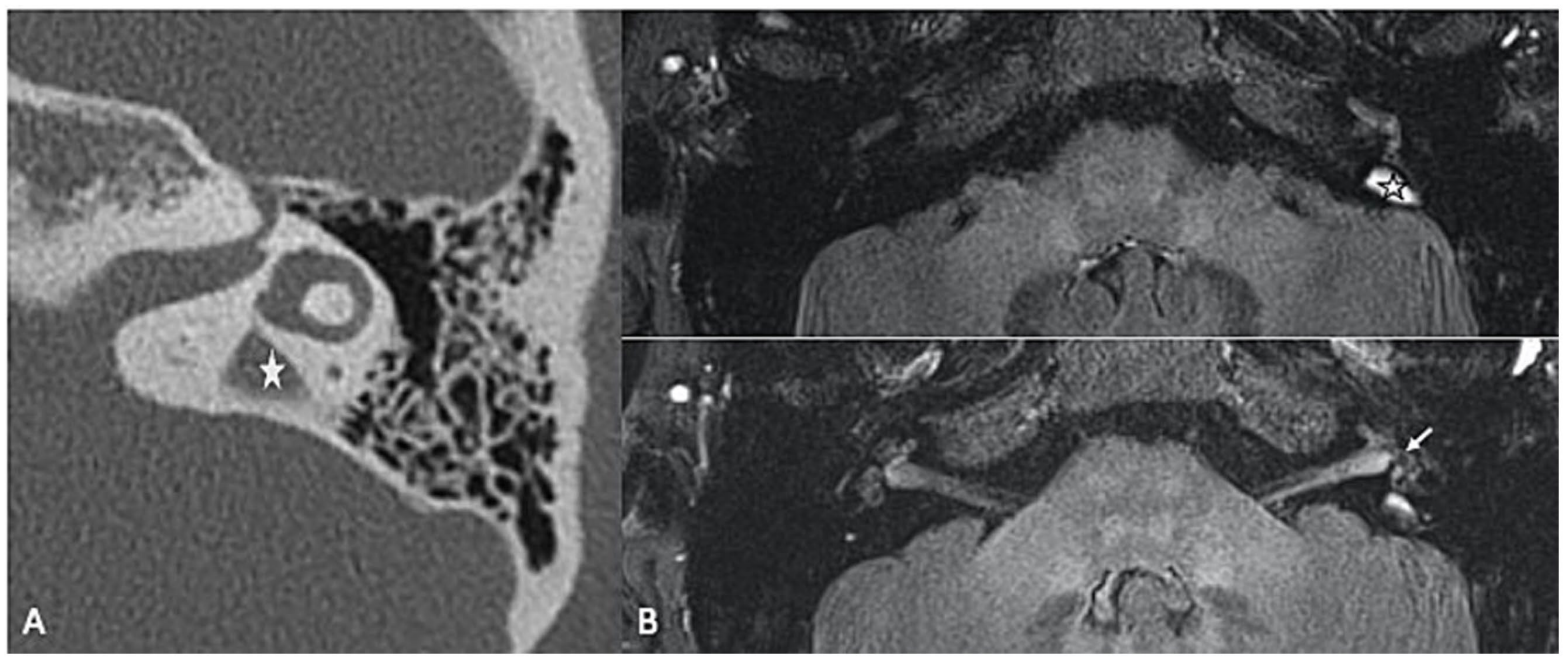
(A) High resolution CT of the temporal bone showing an enlarged vestibular aqueduct on the left (LVAS) (star). (B) 3D TSE FLAIR sequence showing a LVAS (star) together with signs of SHE (enlarged saccule, arrow).
